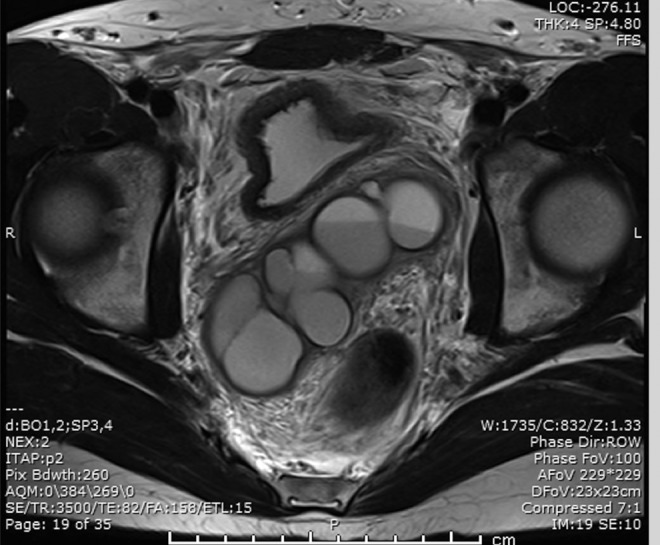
Axial MRI image shows large cystic structure posterior to bladder.
